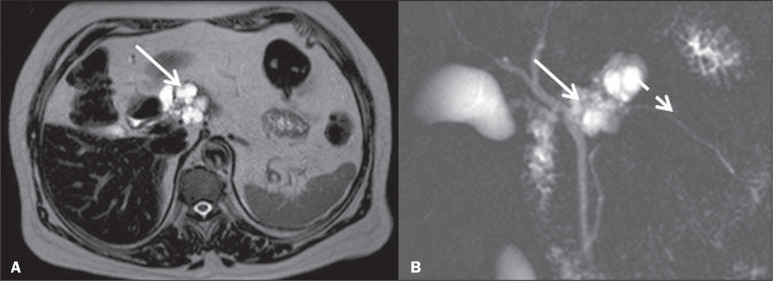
Microcystic adenoma (serous cystadenoma) of the pancreas. T2-weighted
